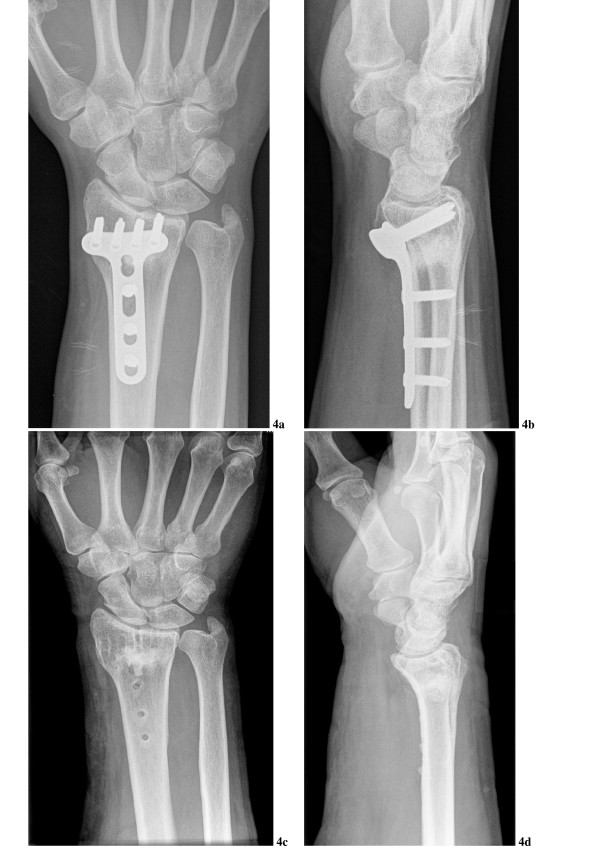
X-ray images of the hand of patient No. 5 three months after the reconstruction (4a and 4b) and after the plate removal 10 months later (4c and 4d). New bone has refilled the metaphyseal defect.
